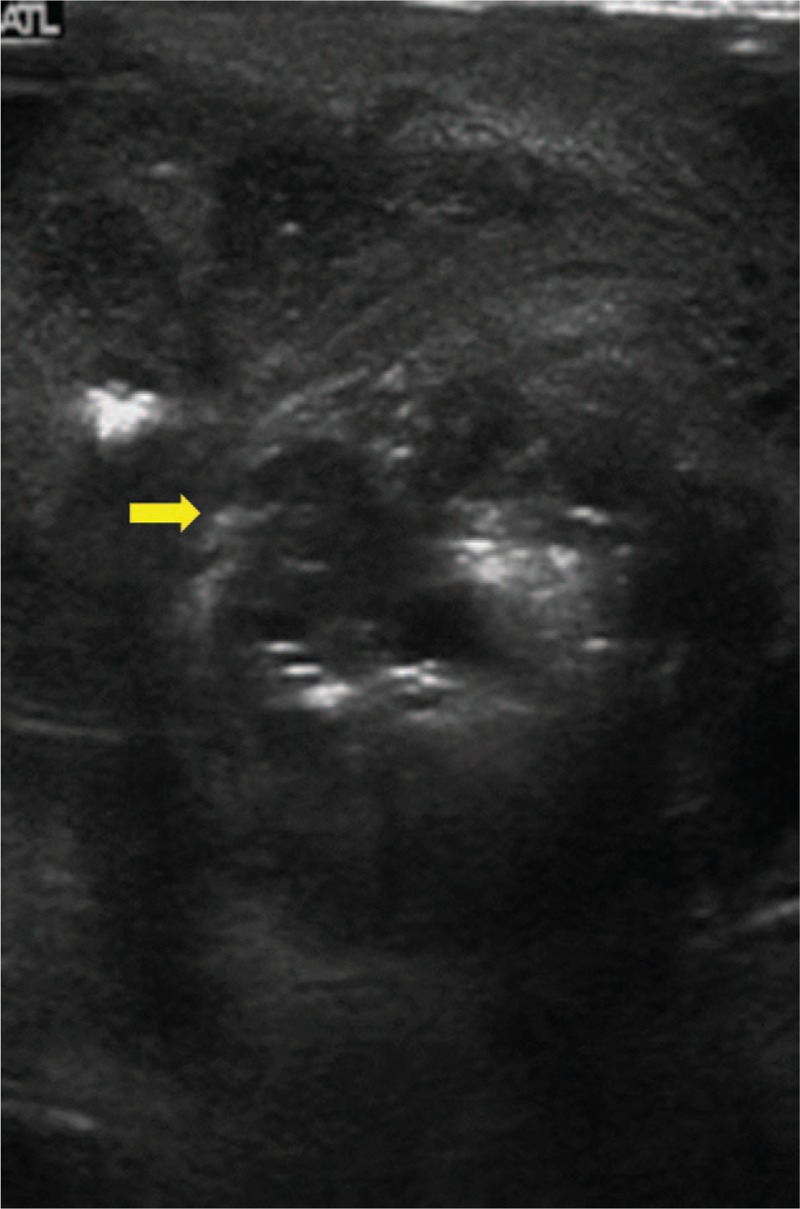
Scrotum sonography showed bright spots and hypoechoic areas.
